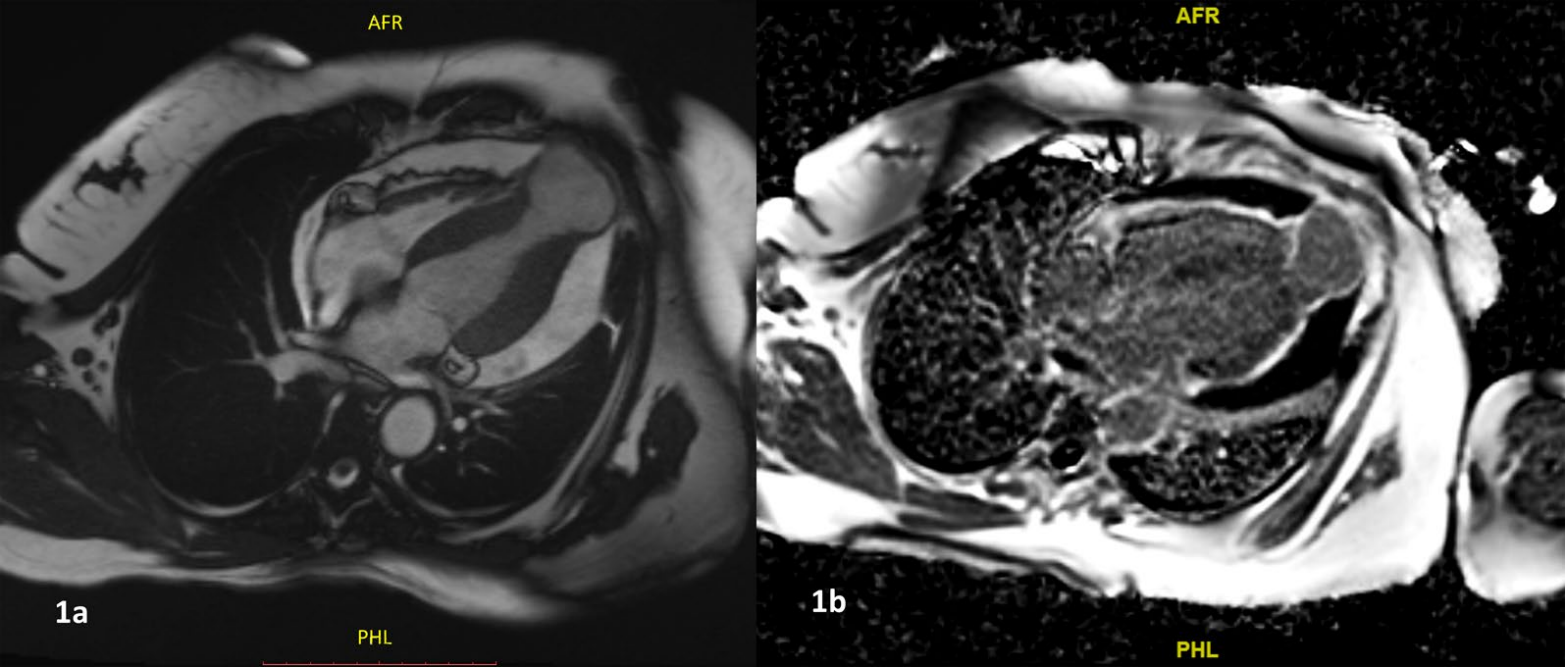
a Axial section of the cardiac MRI showing the left ventricular apical aneurysm and moderate circumferential pericardial effusion. Transmural late gadolinium enhancement of the aneurysmal segment is seen in (b)
